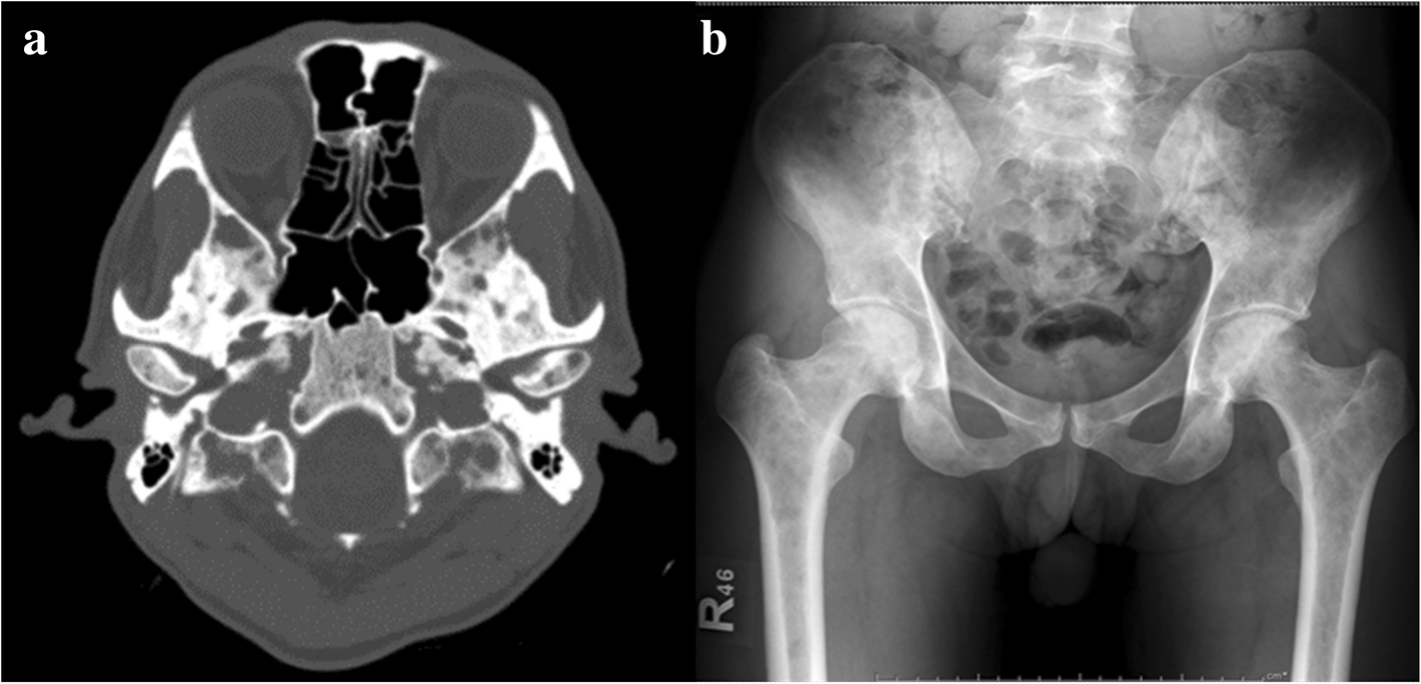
Multiple osteolytic bone lesions on imaging. a Computed tomography of brain, revealing several osteolytic bony destruction at skull base; b film X-ray, displaying multiple osteolytic lesions on pelvis and both femurs
